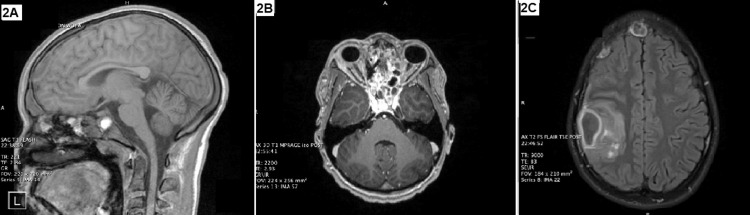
(A-C) Brain magnetic resonance imaging on hospital day 14 revealed a collection overlying the right frontotemporal lobe that increased in size, new surrounding vasogenic edema, and parenchymal enhancement.
